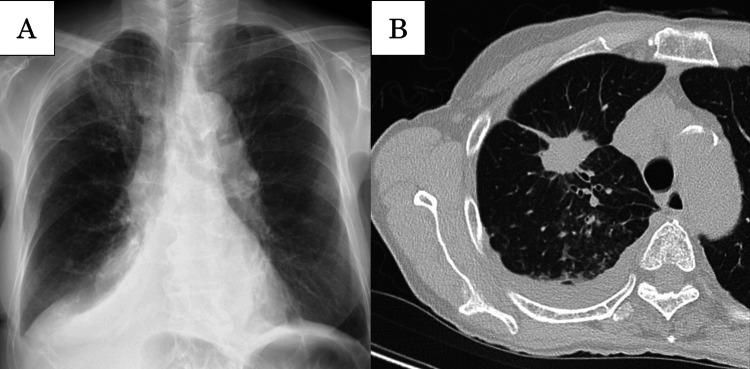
Chest radiography and computed tomography of the chest at admission. (A) Chest radiography demonstrated a ground‑glass opacity in the right upper lung zone, accompanied by a right pleural effusion. (B) Computed tomography of the chest revealed a 26‑mm nodule with irregular margins in the right upper lobe, together with a right pleural effusion.
